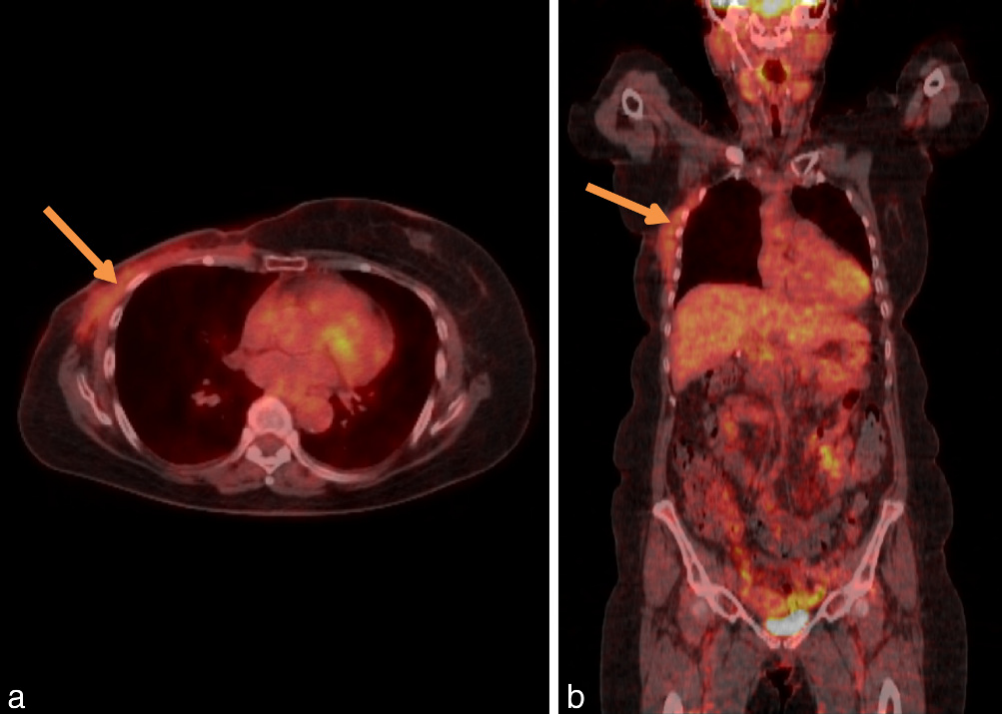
(a) Axial and (b) coronal views ofpost-operative ^18^F-fludeoxyglucose positron emission tomography-CT demonstrating low level metabolic activity at the operative site, likely owingtopost-operative inflammation.
